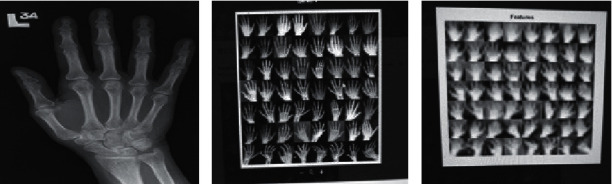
Training of hand X-ray thorough system. (a) Original hand X-ray image. (b) Input images for training. (c) Featured images during training.
